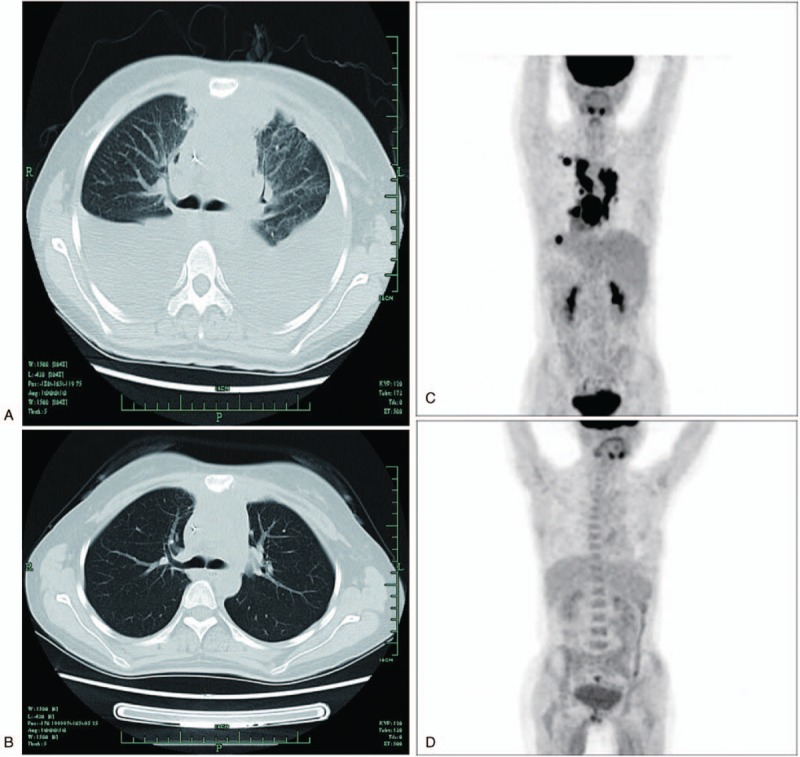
Changes of hydrothorax and masses of lymphoma infiltration after CART19 infusion. (A) Lung CT image on day 7 after CART19 infusion showing bilateral pleural effusion. (B) Lung CT image on day 21 after CART19 infusion showing that the pleural effusion on both sides were completely absorbed. (C) PET/CT before treatment showing high metabolic area in the chest. (D) The patient's chest was clean on day 28 after treatment. CART19 = CD19-directed CAR-T cell, CT = computed tomography, PET = positron emission tomography.
